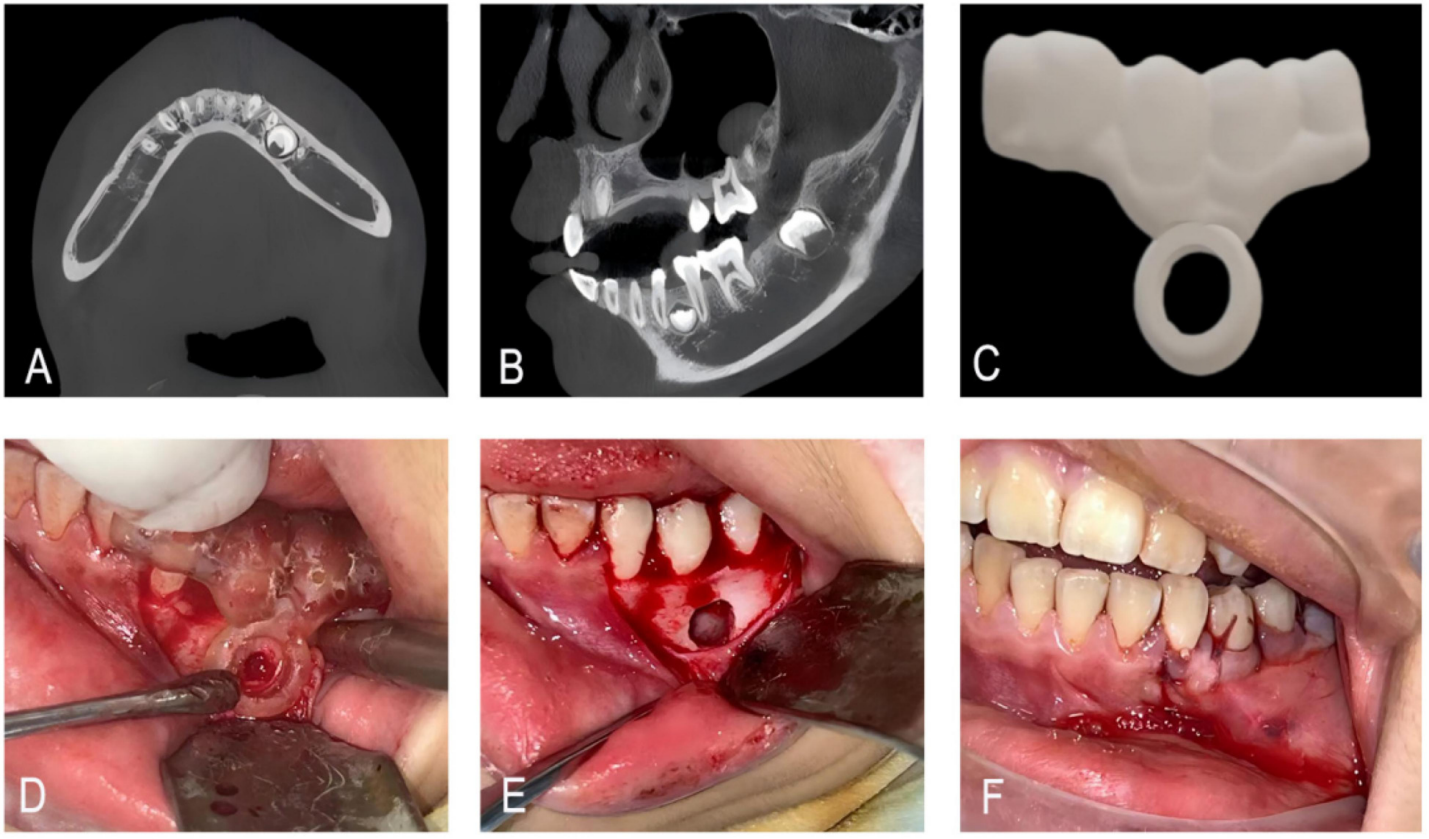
(A,B) CBCT showing a supernumerary tooth apical to the roots of teeth #34 and #35. (C) Guide plate designed by 3D printing. (D) Place the 3D guide plate and remove the bone along the indicator ring. (E,F) Extract the supernumerary teeth and suture the incision.
